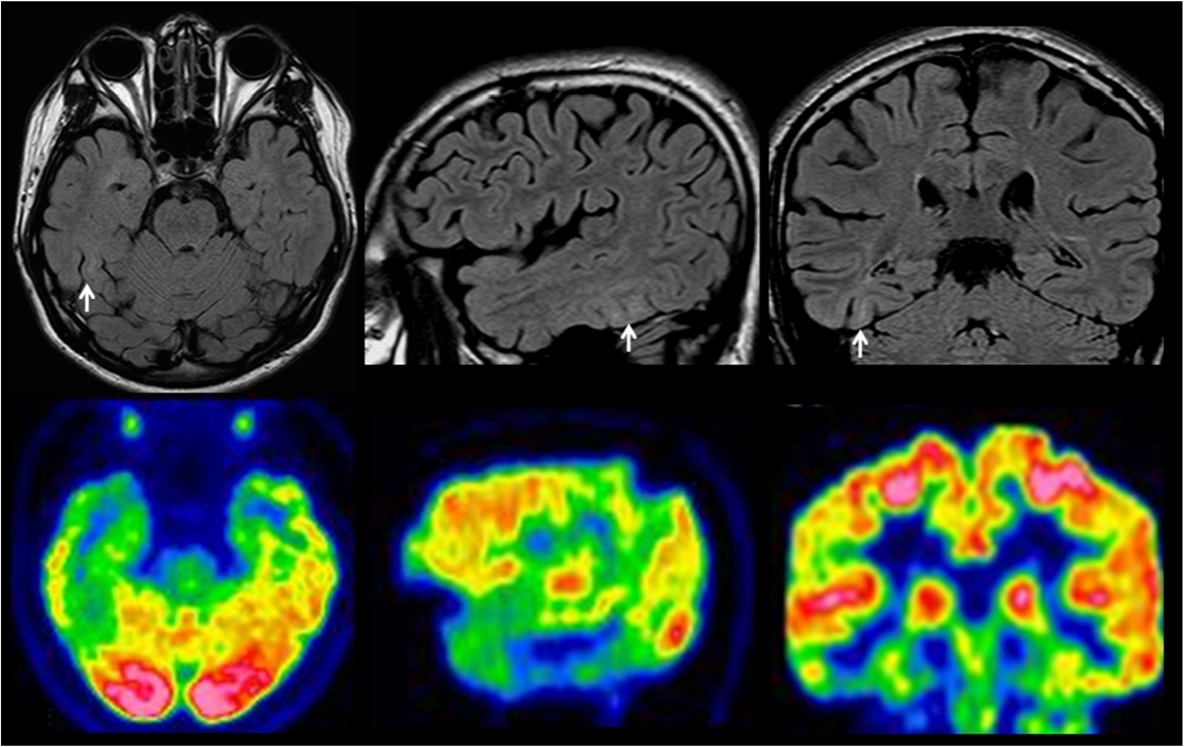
Brain MRI and PET scans of Case 2. T2flair imagins of brain MRI showed the high abnormal signals involving right middle-posterior part of ITG, lateral occipito-temporal sulcus and fusiform gyrus. PET scan demonstrated the hypometabolism regions over inferior and basal aspects of right temporal lobe, as well as the medial temporal structure
